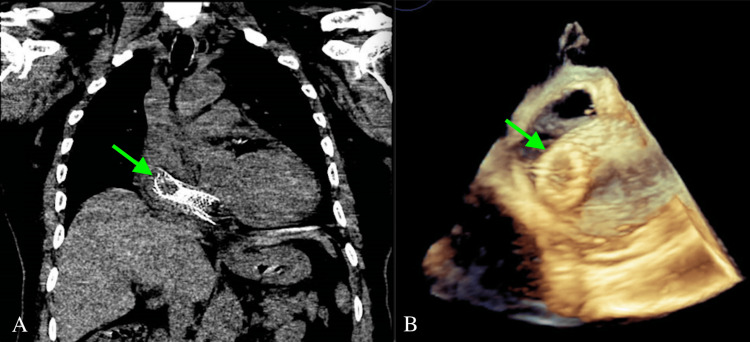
Right Common Iliac Vein Stent Migration to the Right Atrium (A) CT chest and (B) three-dimensional transesophageal echocardiogram (TEE) indicating stent migration.
